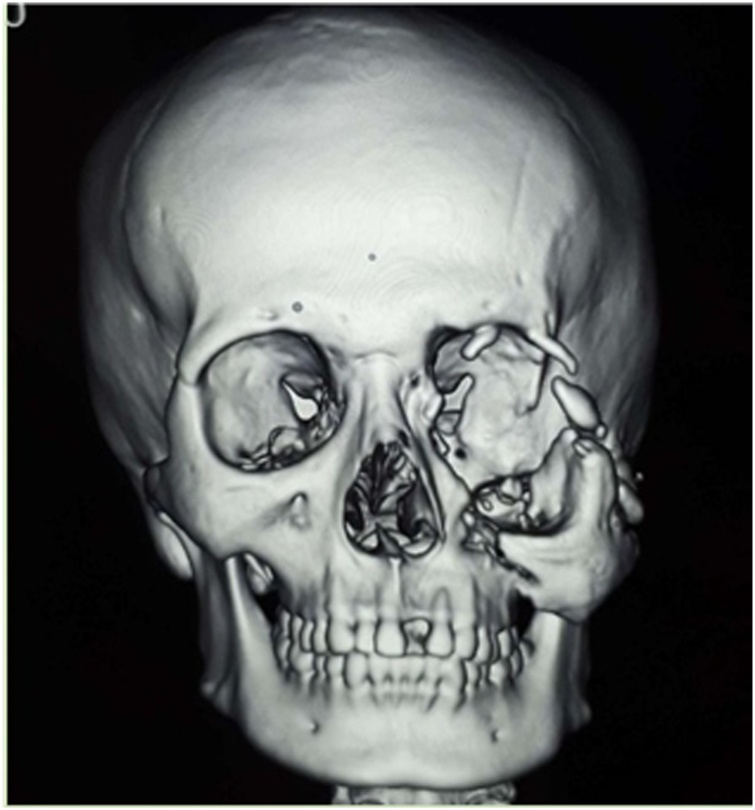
Three-dimensional CT-scan before reconstrucrion.
